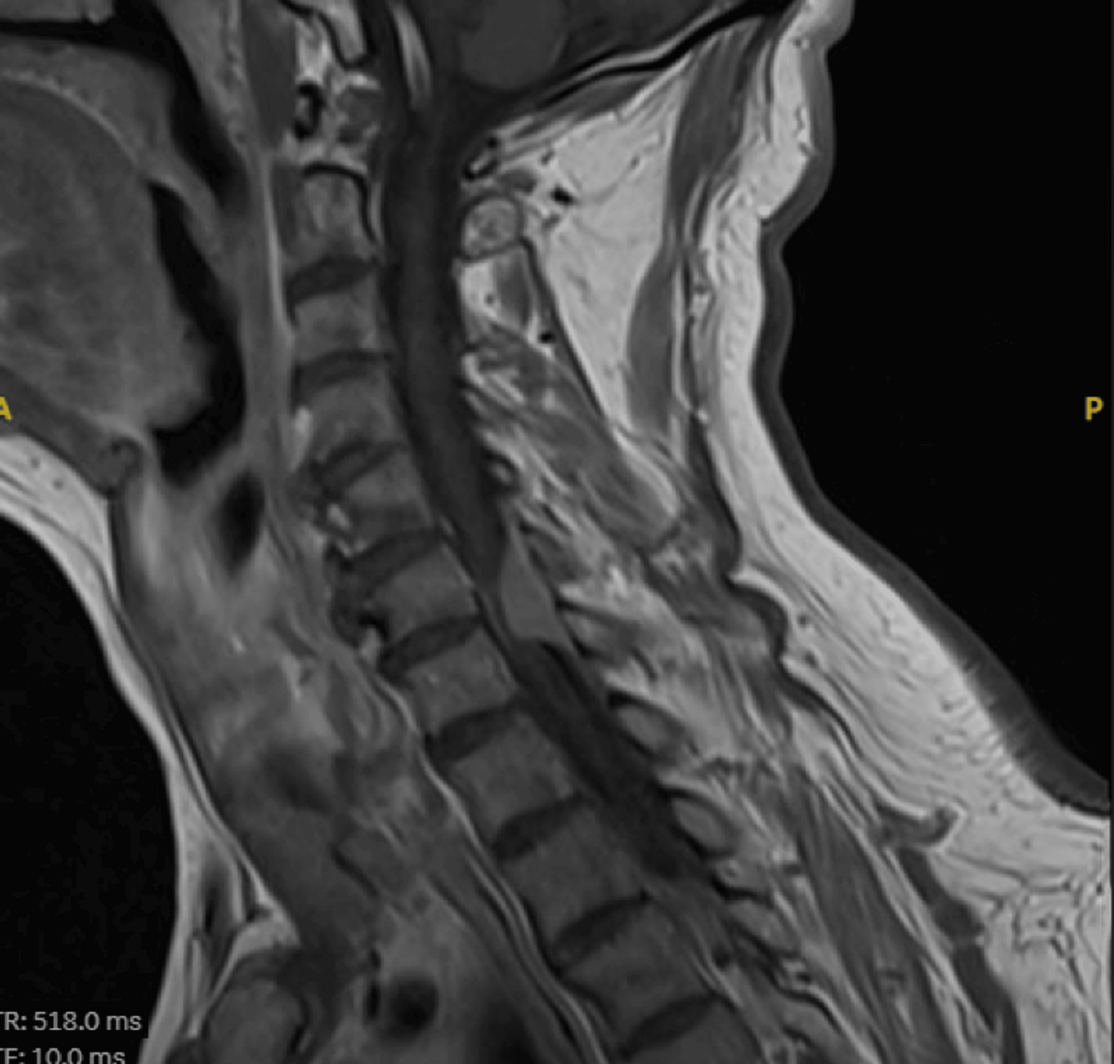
Preoperative cervical sagittal MRI of the patient demonstrated intradural extramedullary lesion at level of C6-C7 causing cord compression.
